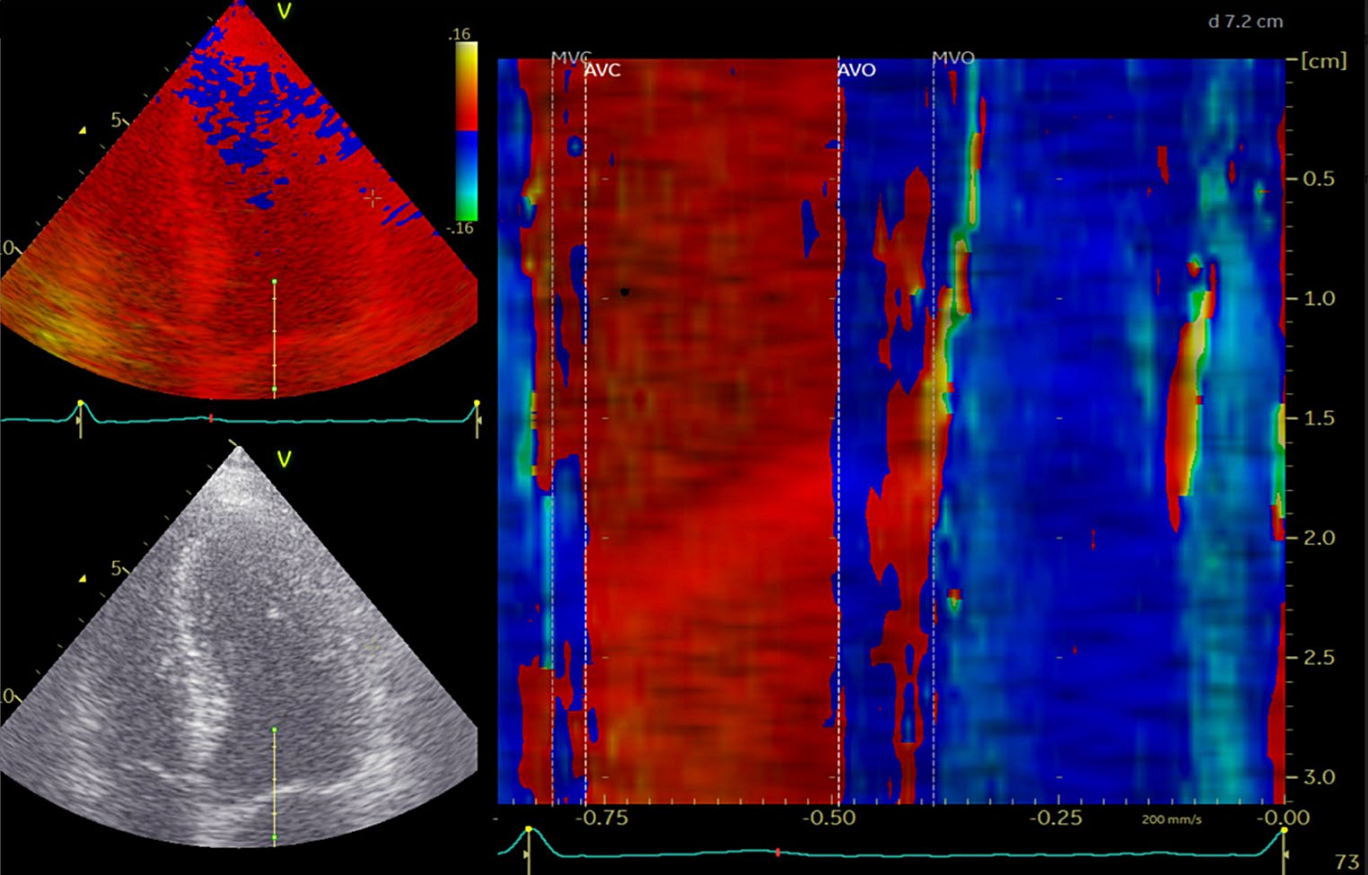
Cardiac time interval measurement. The cardiac time intervals assessed by color tissue Doppler imaging (TDI) M-mode through the mitral leaflet. *Abbreviations* AVO; aortic valve opening, AVC; aortic valve closing, MVC; mitral valve closing, MVO; mitral valve opening
